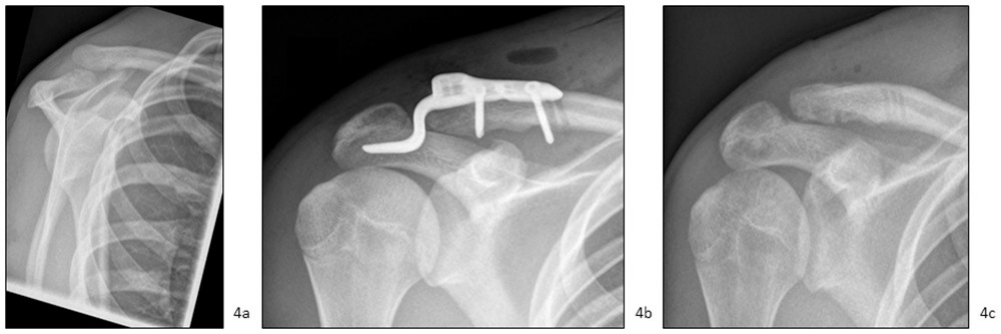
Acromioclavicular joint dislocation treated with hook plate. Preoperative and postoperative x-rays of an acromioclavicular joint (ACJ) dislocation type Rockwood III (horizontal unstable) with surgical treatment using a hook plate (16-year-old boy, fell while playing soccer, implant removal after nine weeks). a) Preoperative x-rays. b) Postoperative x-rays. c) X-rays after implant removal.
